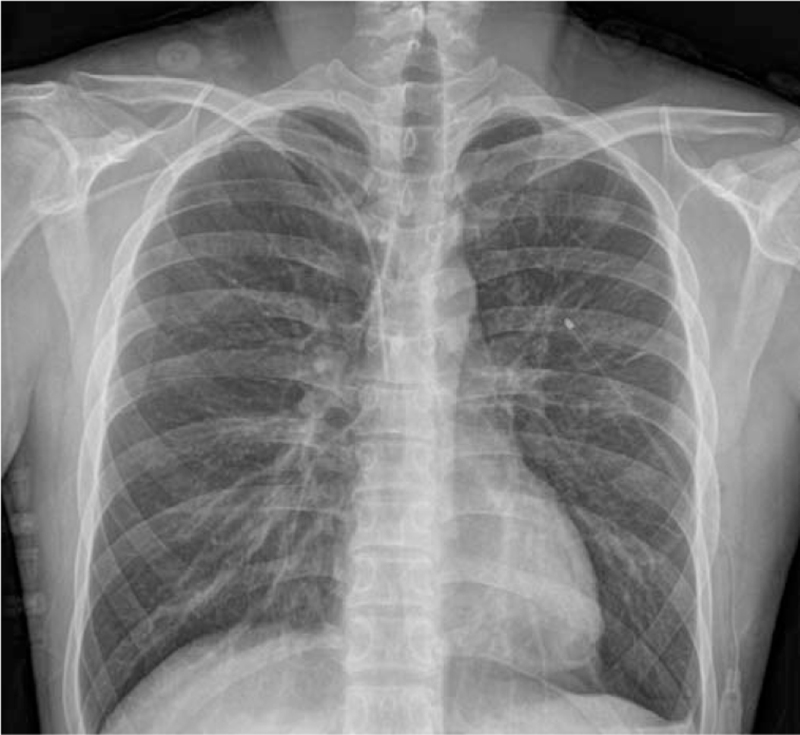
Chest x-ray taken at 6 days after the onset of re-expansion pulmonary edema. The pulmonary edema of the left lung improved.
